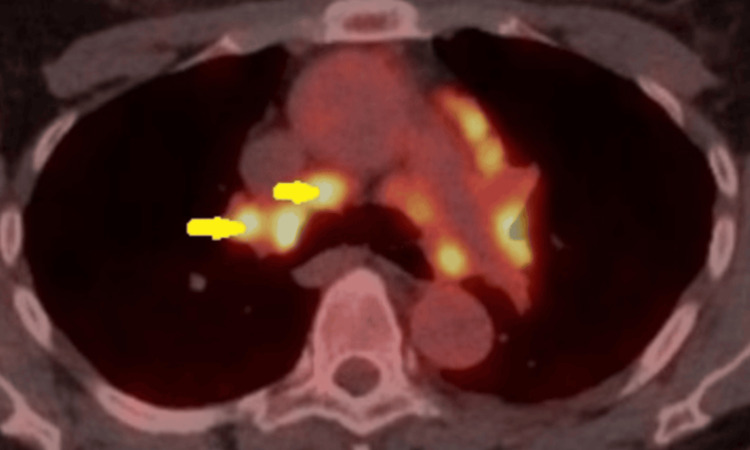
Transverse section of a PET CT study revealing fluorodeoxyglucose (FDG)-avid mediastinal and bilateral hilar lymph nodes PET CT: Positron Emission Tomography Computed Tomography.
